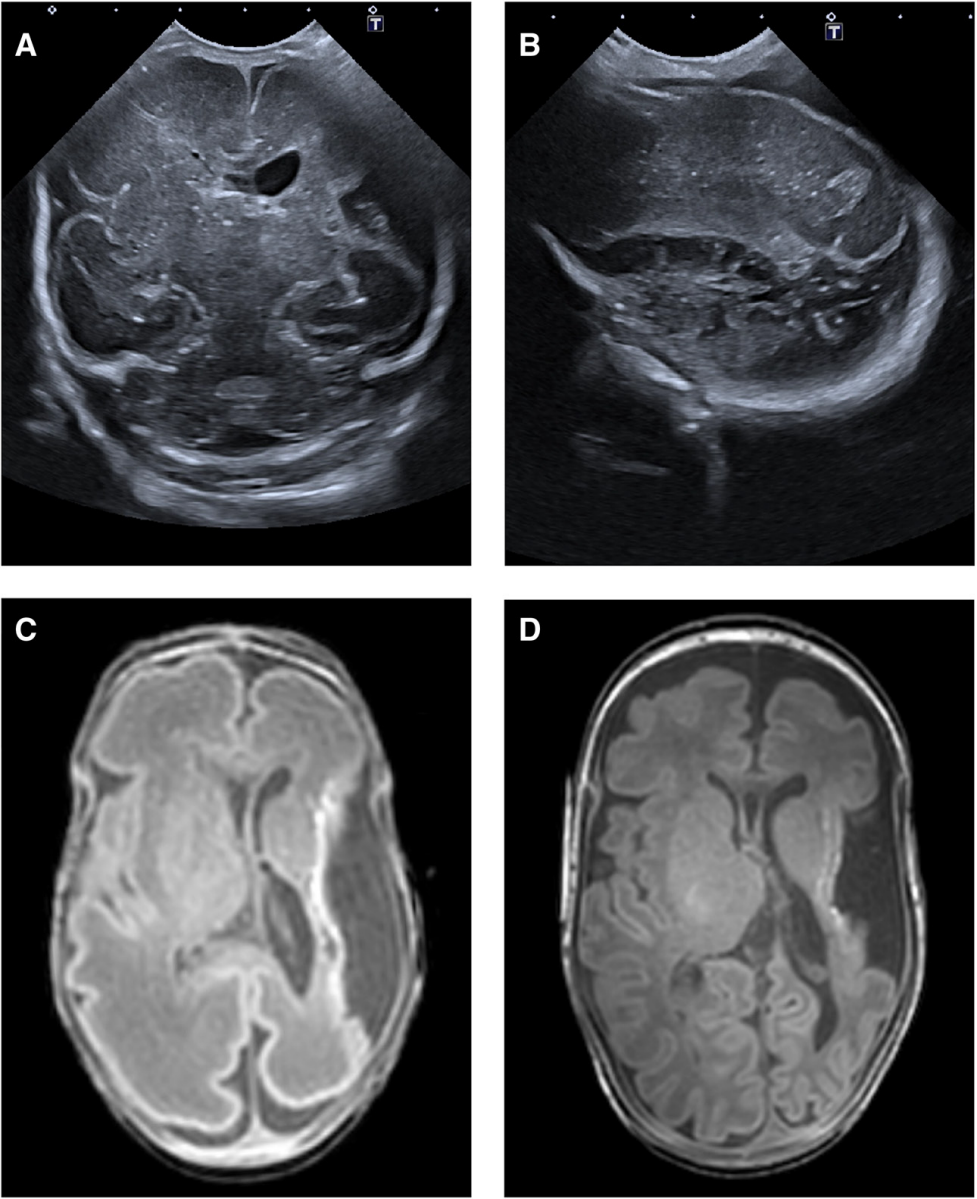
Antenatal arterial ischemic stroke involving left main branch middle cerebral artery (MCA) in an ex-recipient twin with twin-to-twin transfusion syndrome, treated with complete fetoscopic laser surgery (twin 4). A, Cranial ultrasound (cUS; coronal plane) at postnatal day 1 showing tissue loss in the left hemisphere and left lateral ventricle dilatation. B, cUS (sagittal plane) on the same day showing extensive cystic lesions within the parenchyma of the left hemisphere. C, Axial T1-weighted magnetic resonance imaging (MRI) at postnatal day 34 confirming a left main branch MCA (stroke) with ipsilateral ventriculomegaly, loss of cortex, and white matter abnormalities adjacent to operculum and insular region. D, Axial T2-weighted MRI at term-equivalent age demonstrating asymmetrical myelination of the posterior limb of the internal capsule. This infant subsequently developed unilateral spastic cerebral palsy (level 1) at 2 years of age (Table S2—twin 4).
